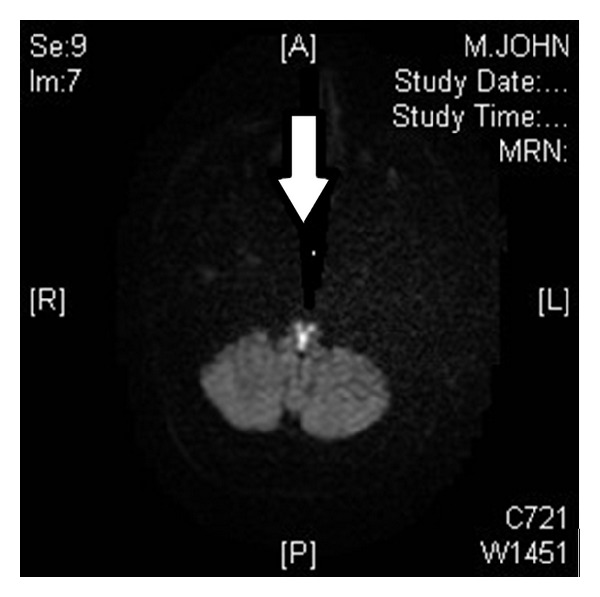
Second brain MRI, DWI shows characteristic “heart appearance” sign suggestive of bilateral medial medullary infarct.
